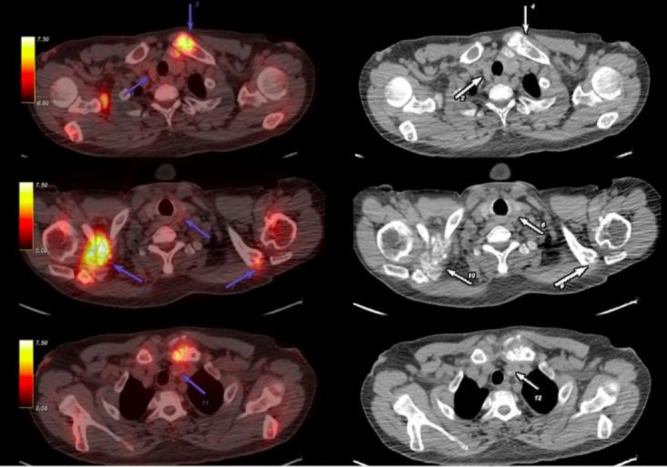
Axial fused PET/CT slices showed three soft tissue density nodules in both tracheoesophageal grooves (two of them located posterior to upper and lower left thyroid lobes and one posterior to right lower thyroid lobe) without significant [^18^F]-FDG uptake (SUV_max_ up to 1.8) measuring up to 20×25mm in left side and 20×10mm in right side, consistent with parathyroid adenomas/hyperplasia. Also multiple bone lesions are noted which are most likely lytic in bilateral clavicles (SUV_max_ up to 6.9 in left clavicle) and bilateral scapulae, more prominent in right side (SUV_max_ up to 9.9) as shown in images
